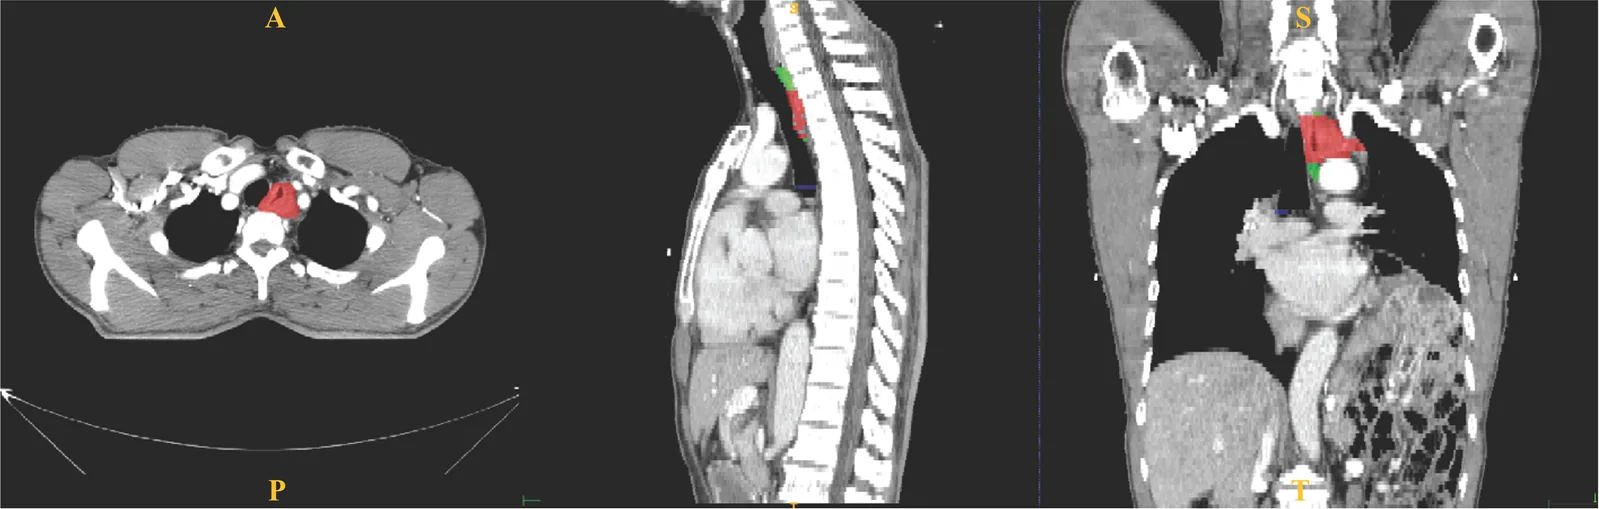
Examples of CT images and tumor lesion contours.
